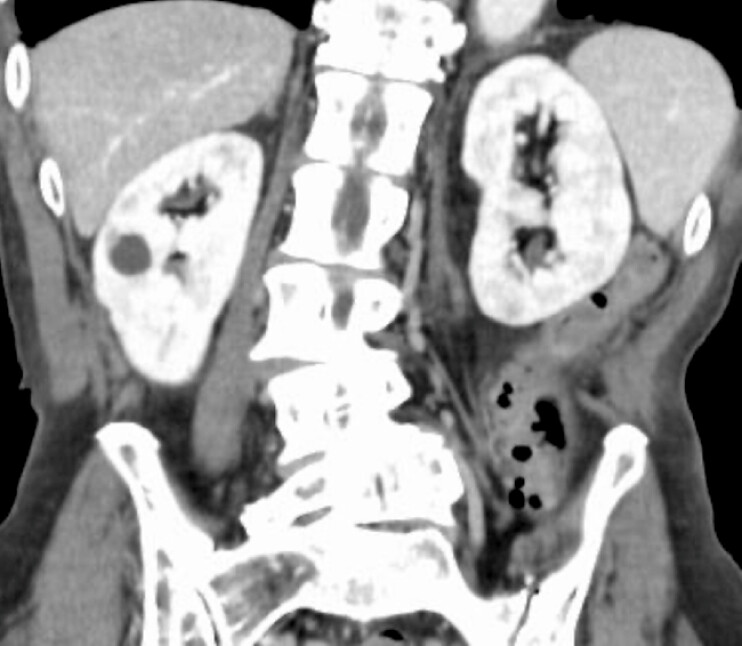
Follow-up CT 2 months later confirmed the resolution of the abscess with minor residual intramural air. Abbreviation: CT, computed tomography.
